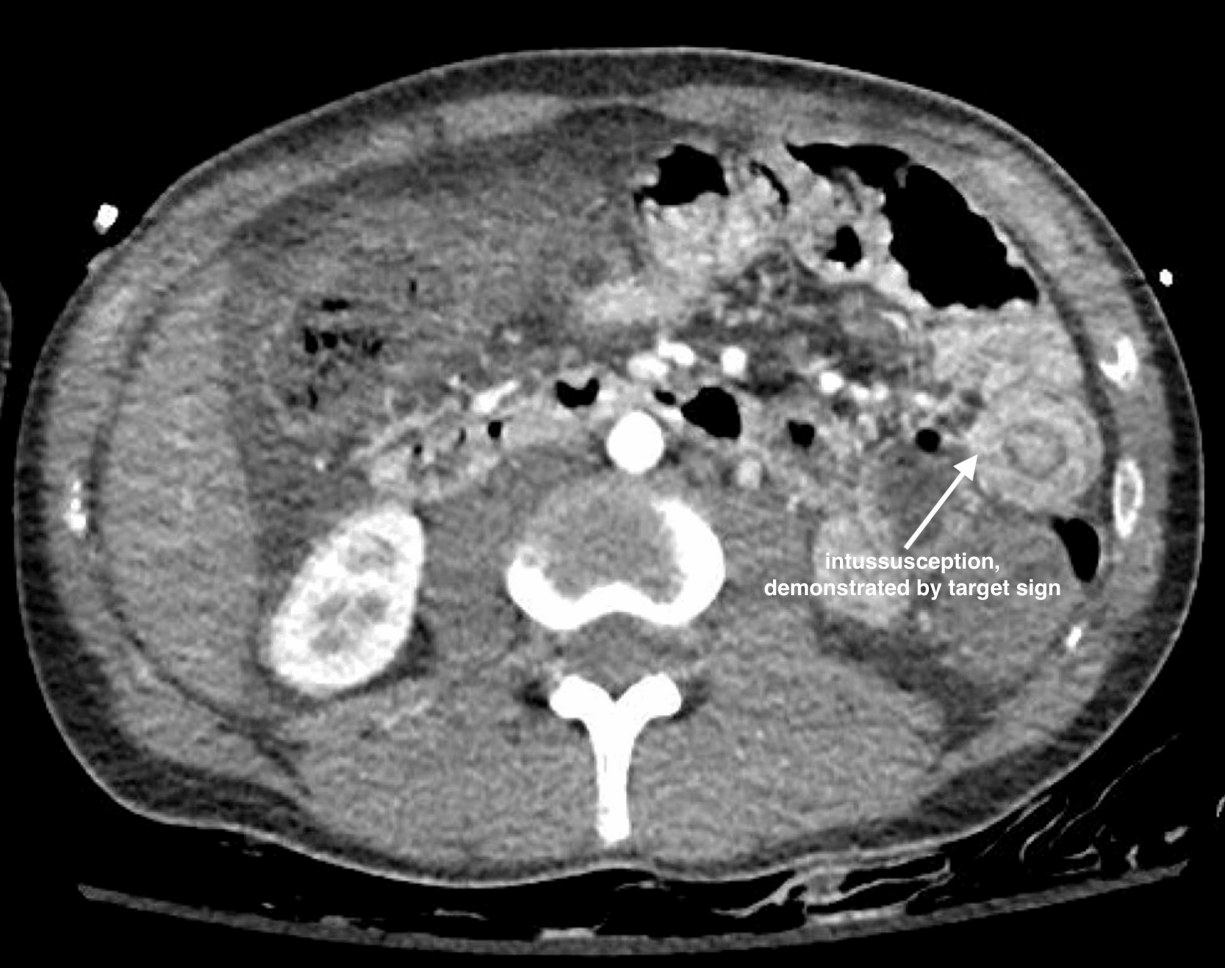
Axial CT angiogram showing intussusception in the left upper quadrant
